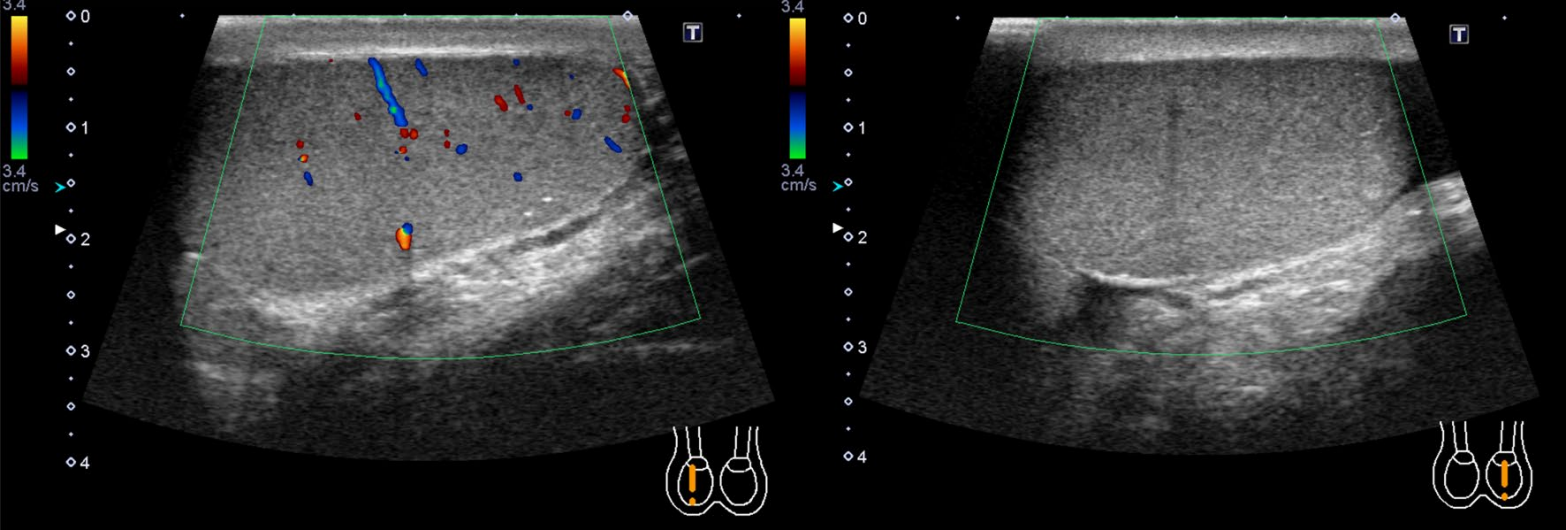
Ultrasound findings. Surface ultrasound scan of the scrotum showed absence of blood flow in the left testis. This finding was suggestive of left testicular torsion
